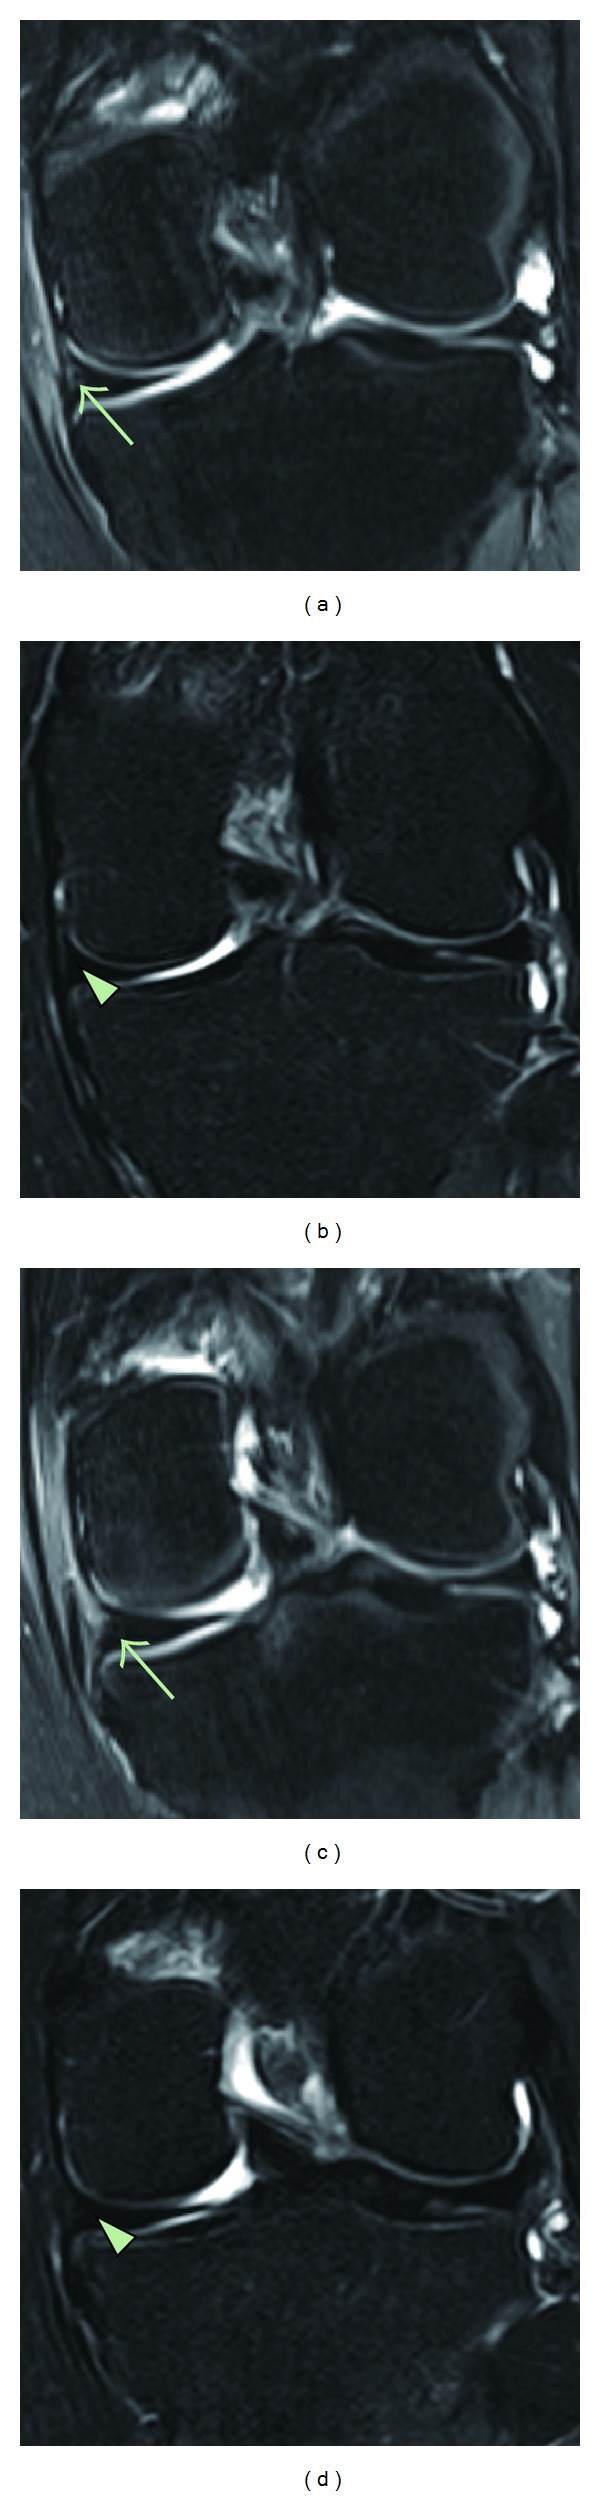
MRI coronal sequential T2 views of the knee. Pretreatment MRI scans ((a) (sequential image: 14/20) and (c) (15/20)) show a tear (arrow) within the posterior horn of the medial meniscus. Posttreatment MRI scans at three months ((b) (14/20) and (d) (15/20)) indicate the healed meniscus (triangle) that has been restored by ASCs mixture-based treatment.
